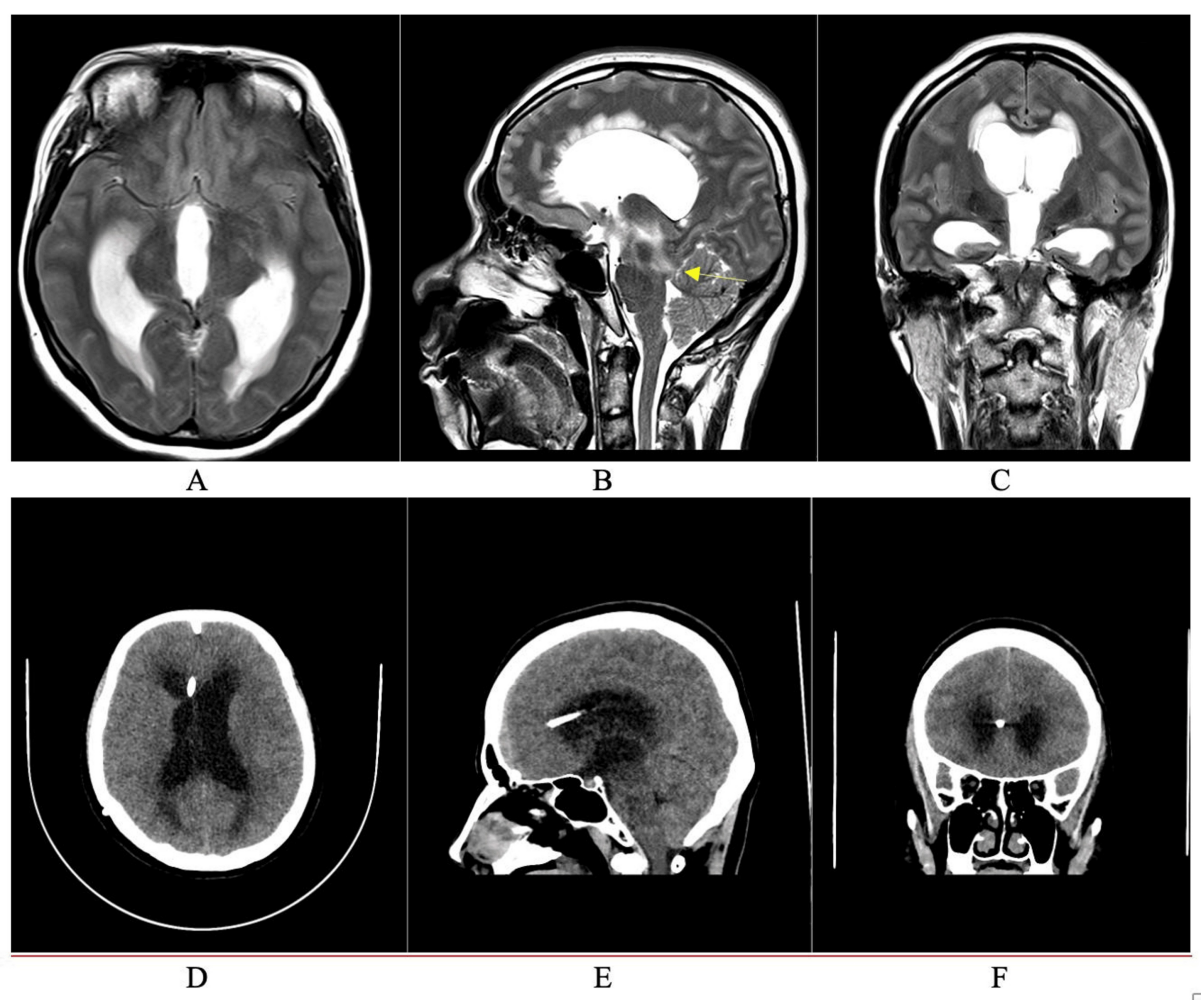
Cranial Imaging (A-C) Cranial MRI T2-weighted images showing obstructive hydrocephalus secondary to an adult-onset aqueductal stenosis (yellow arrow). A: Axial, B: Sagittal, C: Coronal, D-F: Axial, sagittal, and coronal cranial CT images, showing recurrence of the hydrocephalus, and the shunt tip abutting the right frontal lobe. D: Axial, B: Sagittal, C: Coronal.
